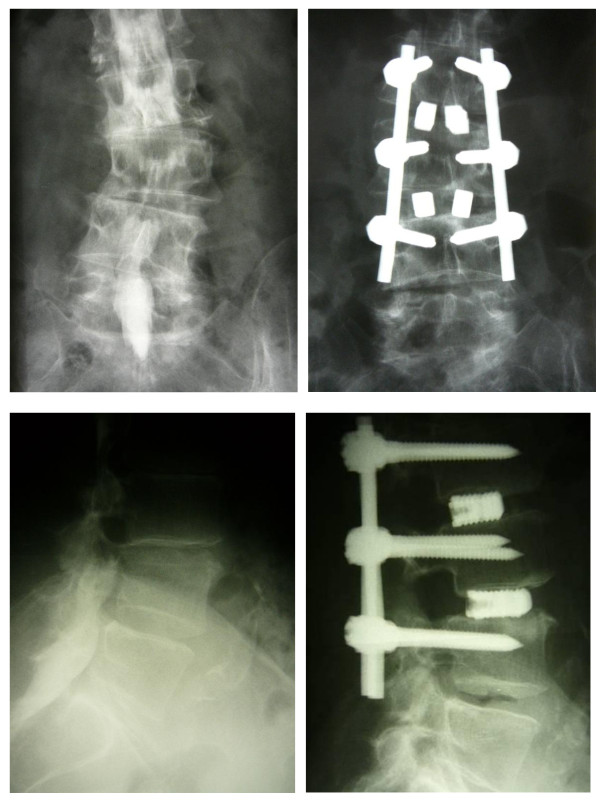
Exemplary pre- and postoperative X-Ray of a degenerative spinal stenosis and scoliosis. 75 years old female patient with degenerative spinal stenosis L2 to L4 and instability. Walking distance of 30 m.
